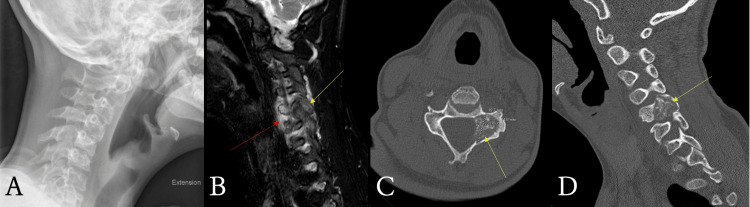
Imaging studies of the cervical spine including (A) radiograph left lateral view without obvious abnormality, (B) MRI sagittal view with T2 hypointense enhancing mass in the C5 pedicle (yellow arrow) with associated marrow edema of the C5 vertebral body and surrounding paraspinal soft tissue edema (red arrow), and CT without contrast (C) axial view measuring an osseous mass of the C5 left pedicle (yellow arrow) at 19.7 mm craniocaudally x 14.9 mm AP and (D) sagittal view showing a mass of the C5 pedicle (yellow arrow) with severe C5-C6 foraminal narrowing AP: anteroposterior
